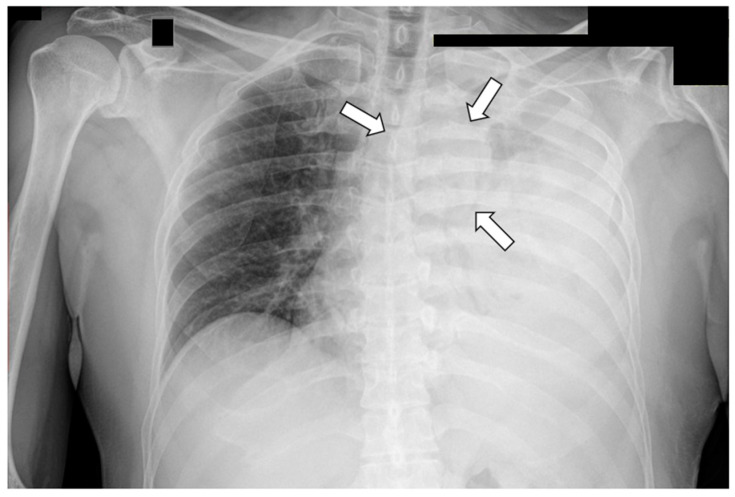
X-ray image; Initial diagnosis of parapneumonic effusion in a previously healthy middle-aged male with chest discomfort and limiting dyspnoea. A careful inspection of the CXR identifies a solid paratracheal area raising concerns of neoplasia (white arrows).
